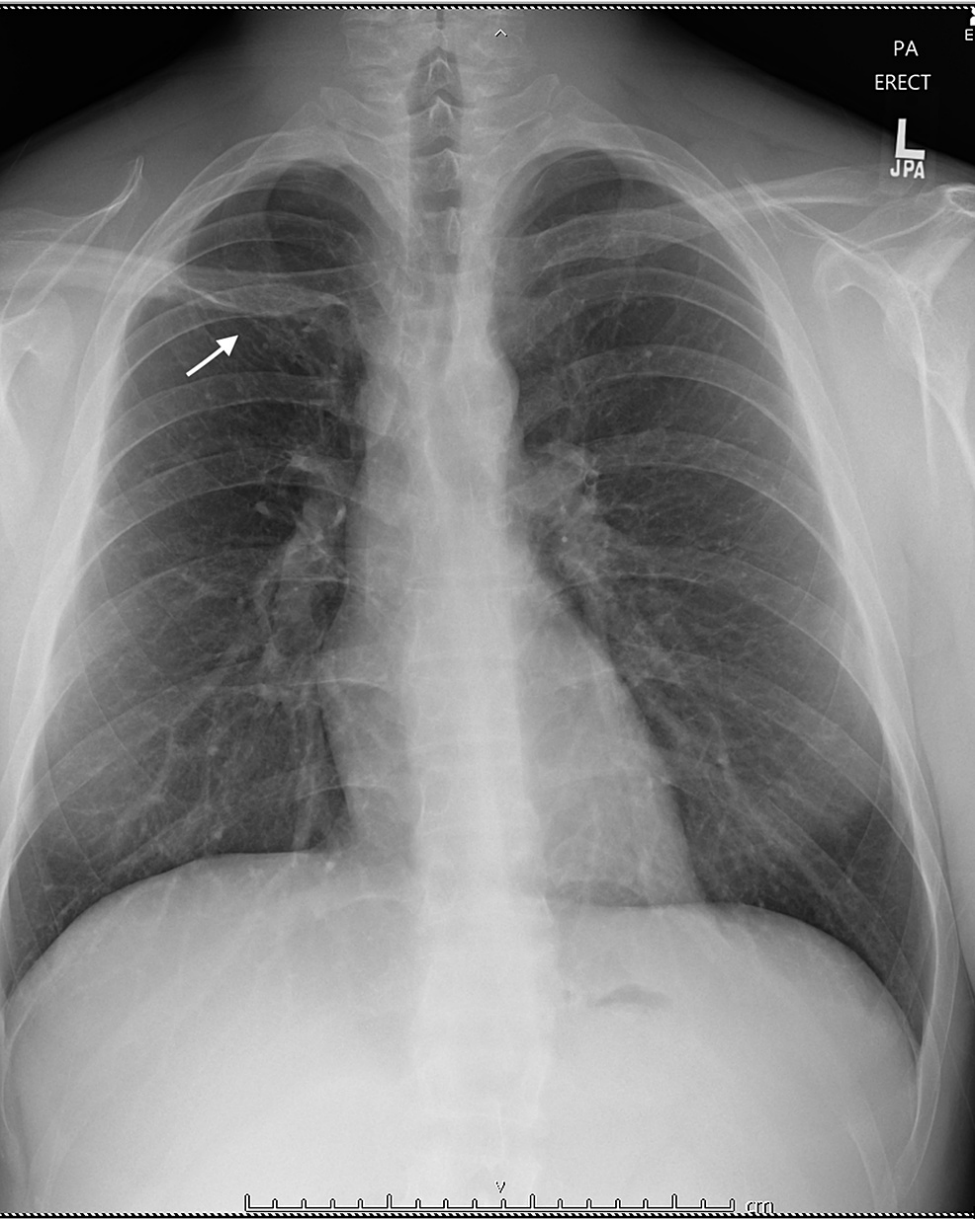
Chest X-ray. Linear scarring or subsegmental atelectasis in the right upper lobe.
